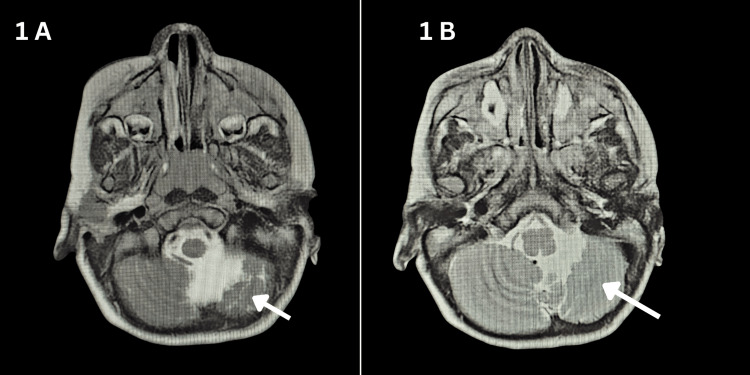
Axial T2-weighted brain MRI showing left cerebellar hemiatrophy. (A, B) Selected posterior fossa axial T2-weighted images demonstrate relative volume loss of the left cerebellar hemisphere compared with the right side, consistent with chronic left cerebellar hemiatrophy. The imaging findings support a remote ischemic insult and correlate clinically with the patient’s right-sided spastic hemiparesis.
